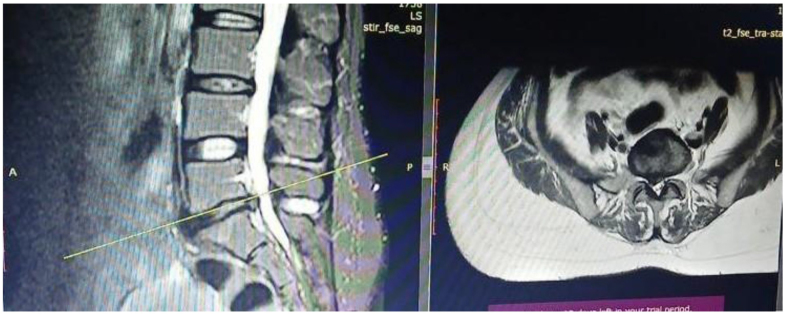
CT scan of case 1 with herniation of lumbar disk with radiculopathy.
